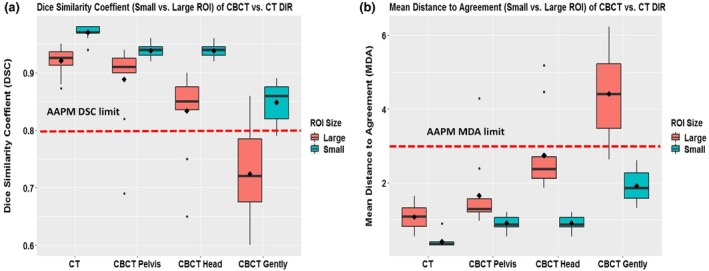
Accuracy of deformable image registration with large and small regions of interest (ROIs) for CT vs CBCT with different imaging protocols. (a) Dice similarity coefficient (DSC) values were higher than the American Association of Medical Physicists (AAPM) limit (red dashed line) for both large and small ROIs in all protocols except CBCT Gently vs CT using large ROIs. (b) Mean distance to agreement (MDA) values were lower than the AAPM limit (red dashed line) for all small ROIs in all protocols.
